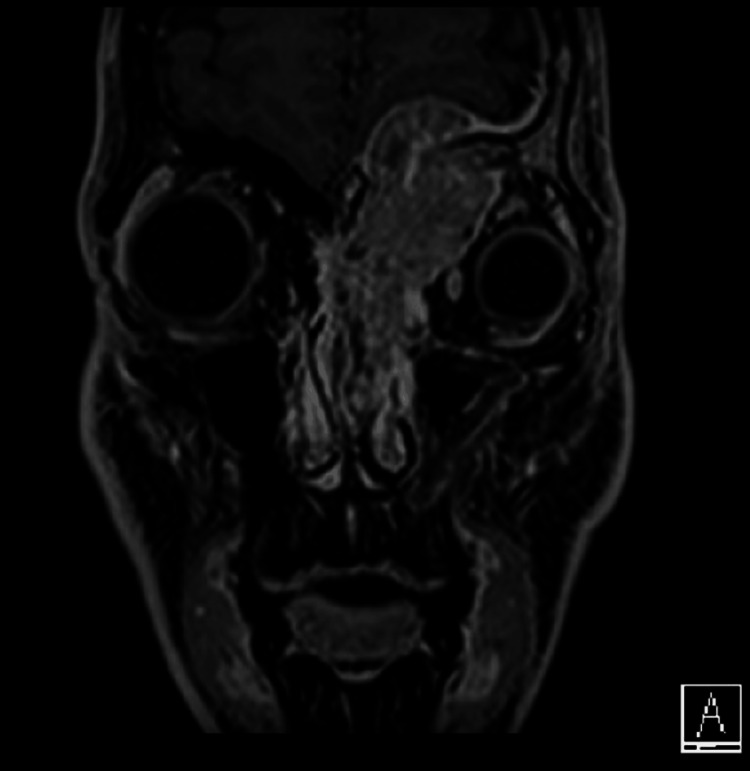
Magnetic resonance imaging (MRI) on admission. The tumor was mainly located in the left ethmoid sinus and filled the nasal cavity. It destroyed the basal skull bone and invaded the dura mater on the cranial side, compressing the brain parenchyma. Additionally, it significantly extended into the left orbit, causing eyeball displacement.
